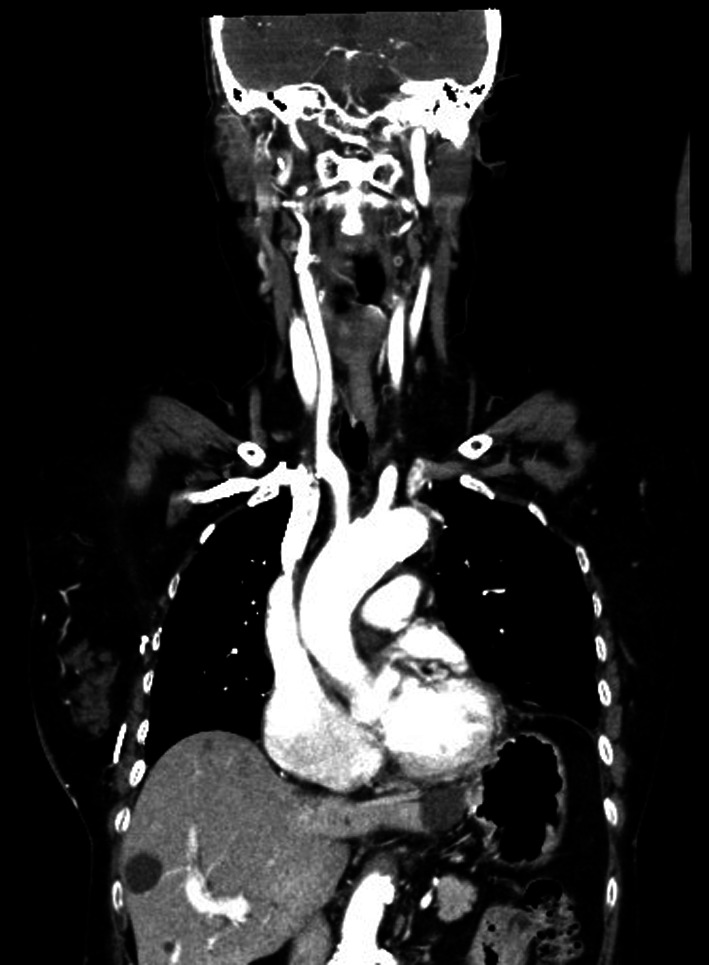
Postoperative contrast-enhanced computed tomography shows no mass in the superior vena cava or right atrium
